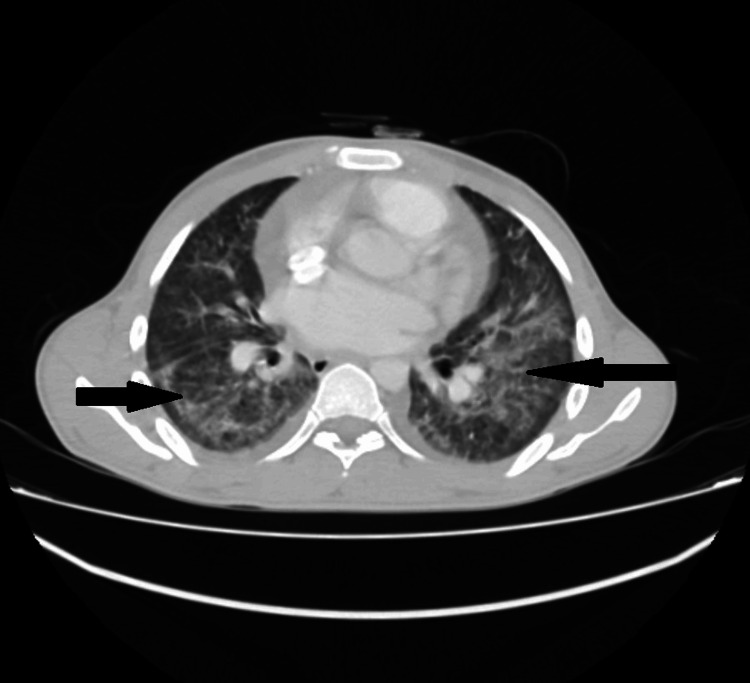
High-resolution CT scan showing bilateral ground-glass opacification, small cavitations, and multiple sub-centimeter mediastinal lymph nodes. Black arrows indicate ground-glass opacities
